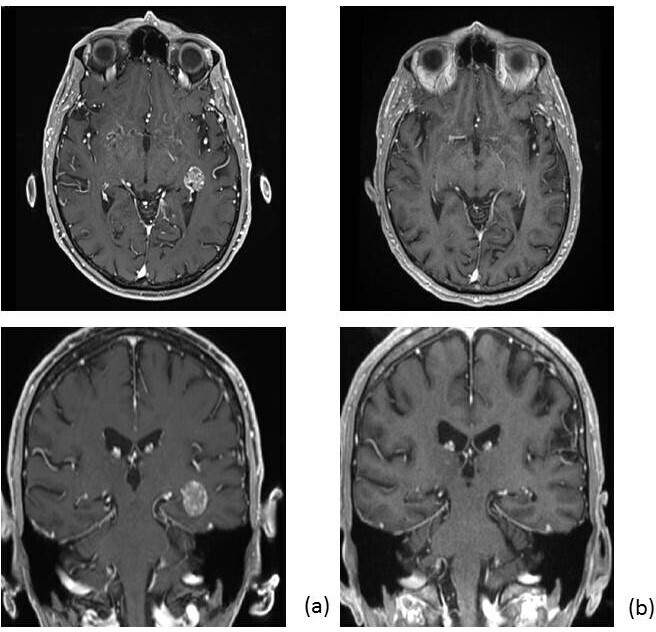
Brain MRI showing a metastases (a) from gastric cancer treated with RS, 24 Gy in 1 fraction with complete response at follow-up MRI (b). RS, radiosurgery.
